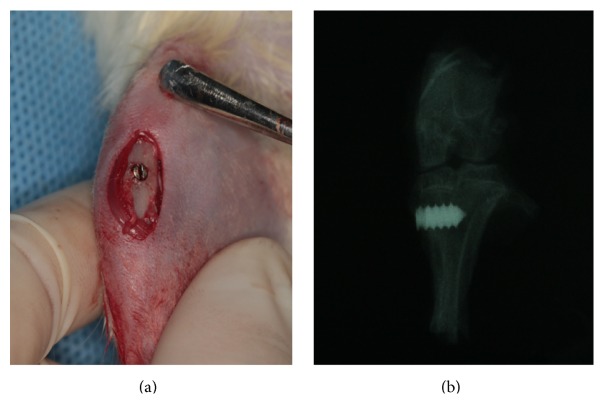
Implant surgery and postsurgery radiograph. (a) The implant was placed into the hole prepared in the tibia metaphysis. (b) Radiograph of tibia with implant after surgery.
